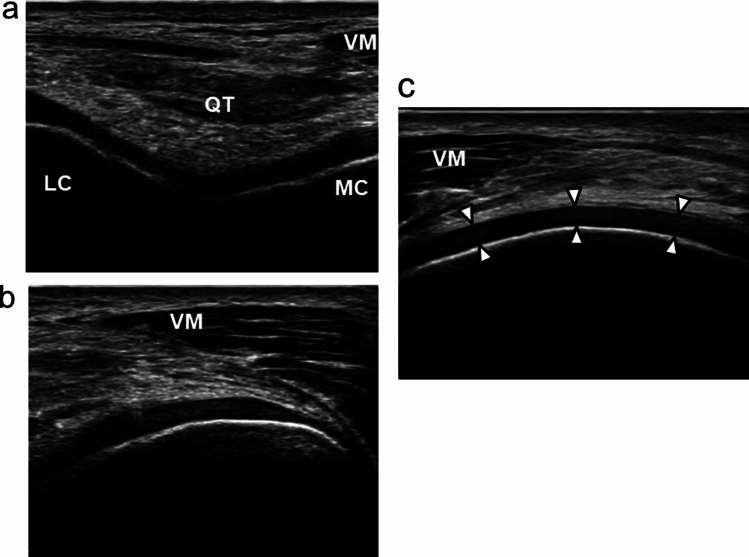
Ultrasound evaluation of cartilage. VM: vastus medialis, QT: quadriceps tendon, LC: lateral condyle, MC: medial condyle. The coronal image of femoral condyles at the level of the upper patella (a), the center of the medial condyle in the image (b), and cartilage on a longitudinal image (c). The arrows at the middle indicate the cartilage thickness
